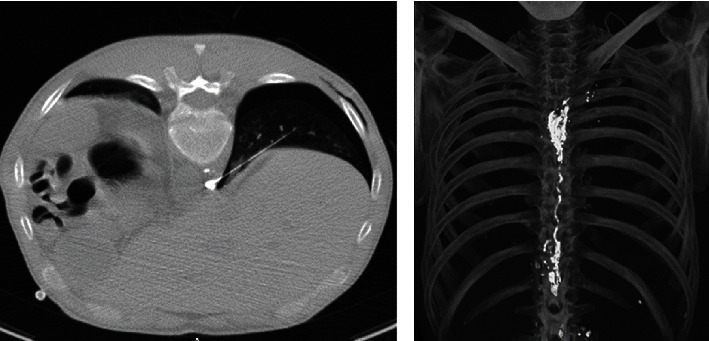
CT confirmation of the fistula site embolization and closure.
